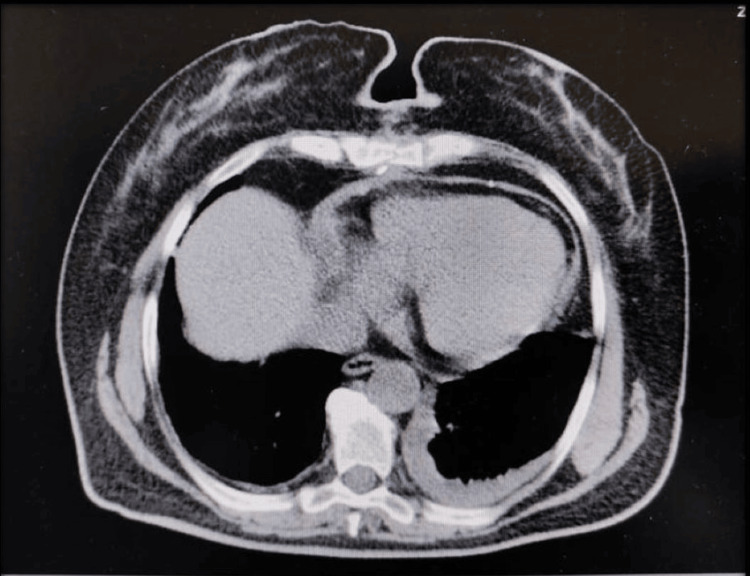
CT-scan performed after the surgical intervention, showing no residual gross mass, and minimal pericardial and pleural fluid
